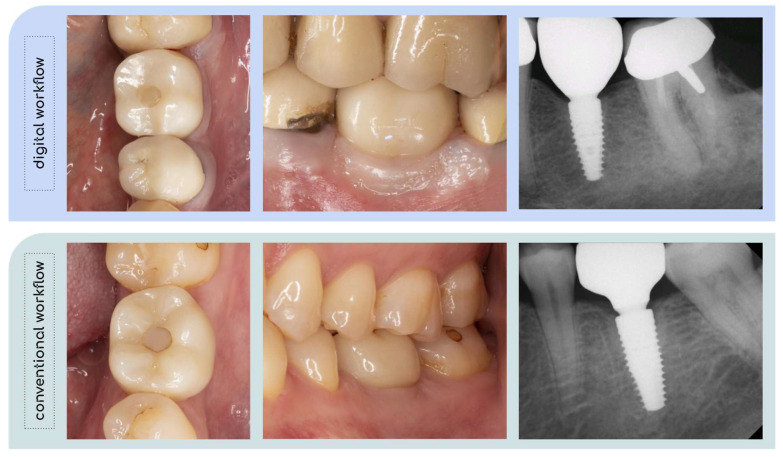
Clinical and radiographic outcomes of two study participants at the last follow-up.
